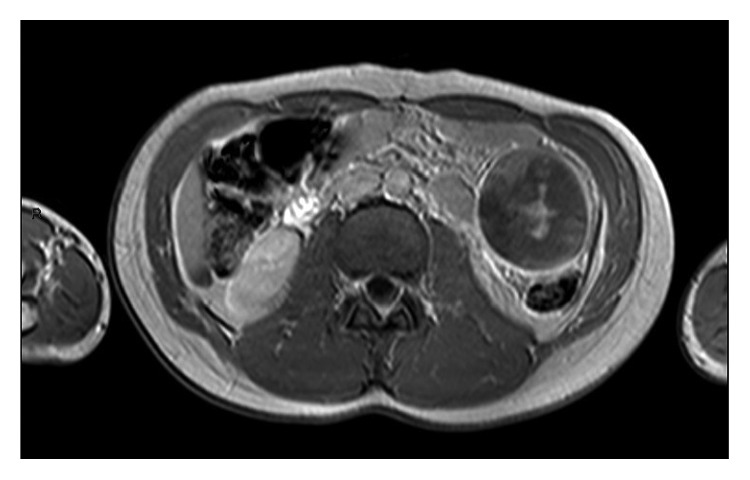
T1-weighted gadolinium-enhanced axial view of MRI showing hemorrhage within the AS.
